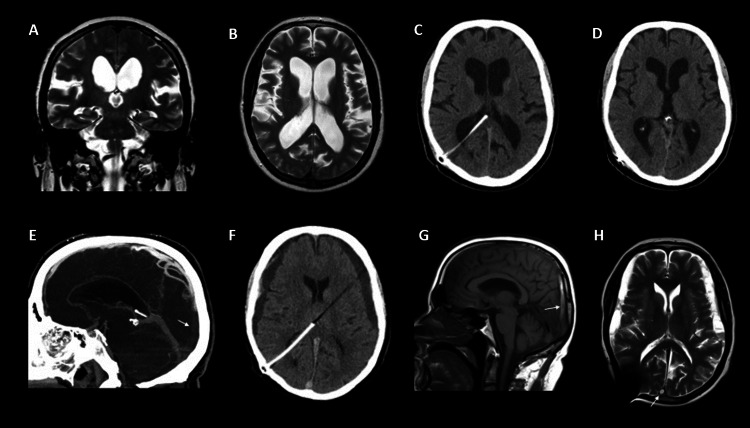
Cerebral venous thrombosis due to overdrainage in a patient with normal pressure hydrocephalus (A) Coronal and (B) axial T2-weighted (T2W) MRI shows ventriculomegaly (Evan’s index superior to 0.3 and acute callosal angle) and disproportionated changes in the subarachnoid spaces (dilated Sylvian fissures and narrow sulci at the high convexity). (C) The first control CT after ventriculoperitoneal shunting reveals correct placement. (D) Three weeks later, a new control CT shows a reduction of the ventricular system. (E) CT venography depicts a filling defect in the posterior superior sagittal sinus (white arrow). (F) Control CT shows bilateral frontal subdural effusions. (G) Midsagittal T1-weighted (T1W) MRI shows a high signal within the posterior superior sagittal sinus consistent with subacute thrombosis (white arrow). (H) Axial T2W MRI reveals slight enlargement and bleeding of subdural collections and a slight hyperintensity within the posterior superior sagittal sinus (white arrow), consistent with thrombosis and partial recanalization of the sigmoid sinus.
